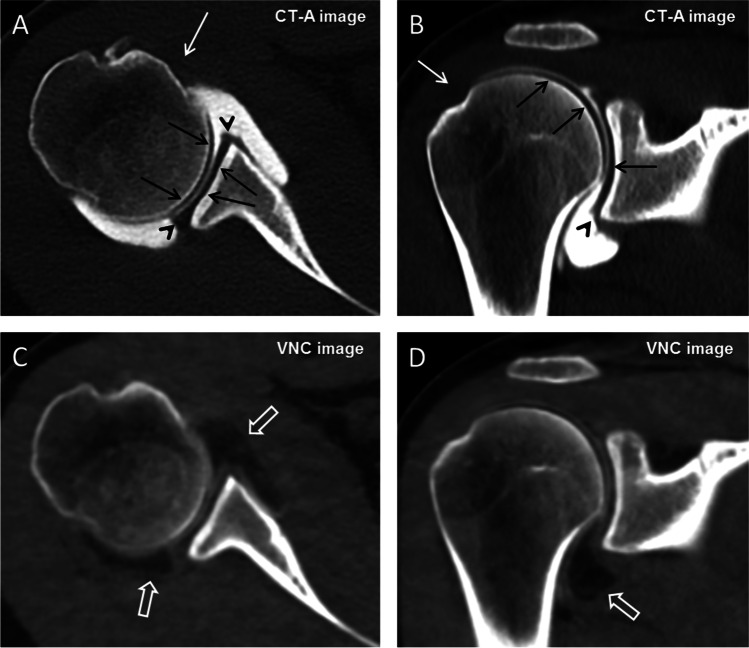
A 20-year-old female with anterior glenohumeral instability. Dual energy CT after arthrography of the right shoulder with reformatted axial (A) and coronal oblique (B) CT arthrogram (CT-A) images clearly show the articular cartilage (black arrows), labrum (black arrow heads), and tendons of the rotator cuff (white arrows). The cortical and trabecular structure of the humeral head and of the glenoid is clearly visible. Reformatted axial (C) and coronal oblique (D) images of the virtual non-contrast (VNC) dataset show full subtraction of the intraarticular iodinated contrast material (open arrows). Note substance loss and fraying of the inferior labrum in (B) because of Bankart lesion. Image contrast between iodine and soft tissue was 1170 Hounsfield units
